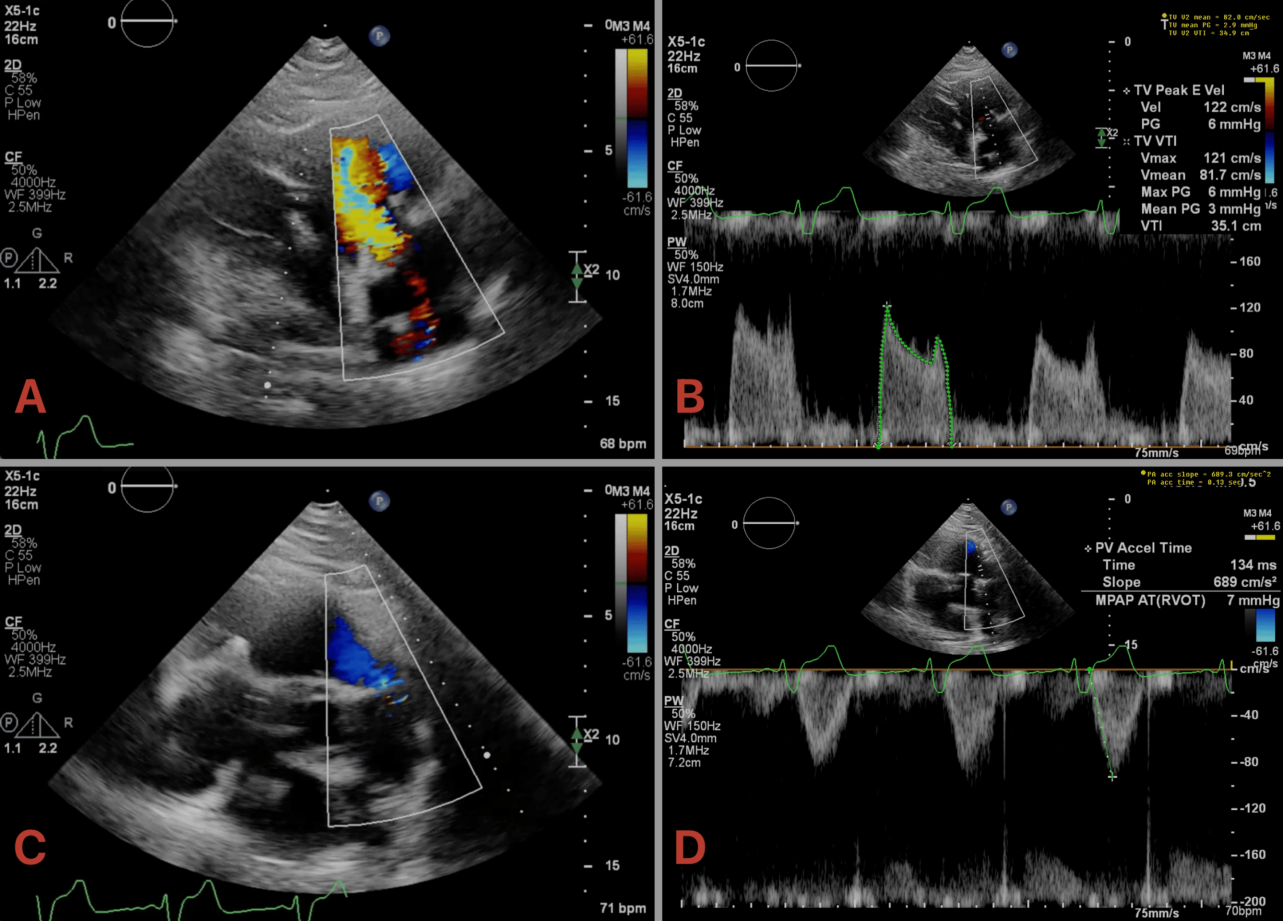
Postoperative transthoracic echocardiography (TTE) demonstrating well-functioning bioprosthesis following dual right-sided valve replacement TTE demonstrating (A) well-functioning bioprosthetic tricuspid valve (TV) with (B) normal gradients (3 mmHg) and (C) normally functioning bioprosthetic pulmonic valve (PV) with (D) normal gradient (7 mmHg).
